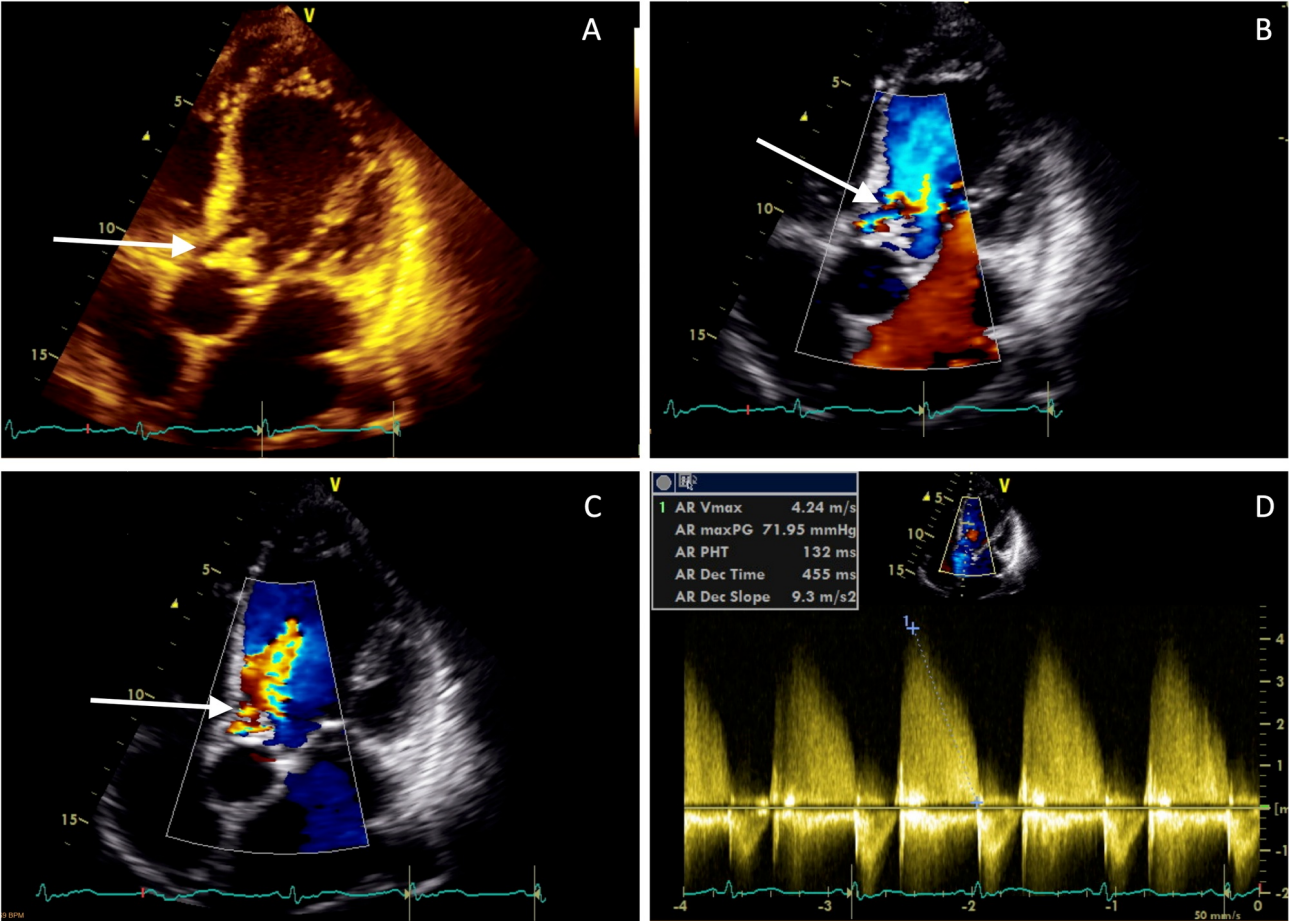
Aortic regurgitation using Doppler imaging. A: Apical five-chamber view displaying the valve perforation B, C: Severe aortic regurgitation using color Doppler flow imaging D: Measurement of aortic regurgitation using spectral Doppler flow
